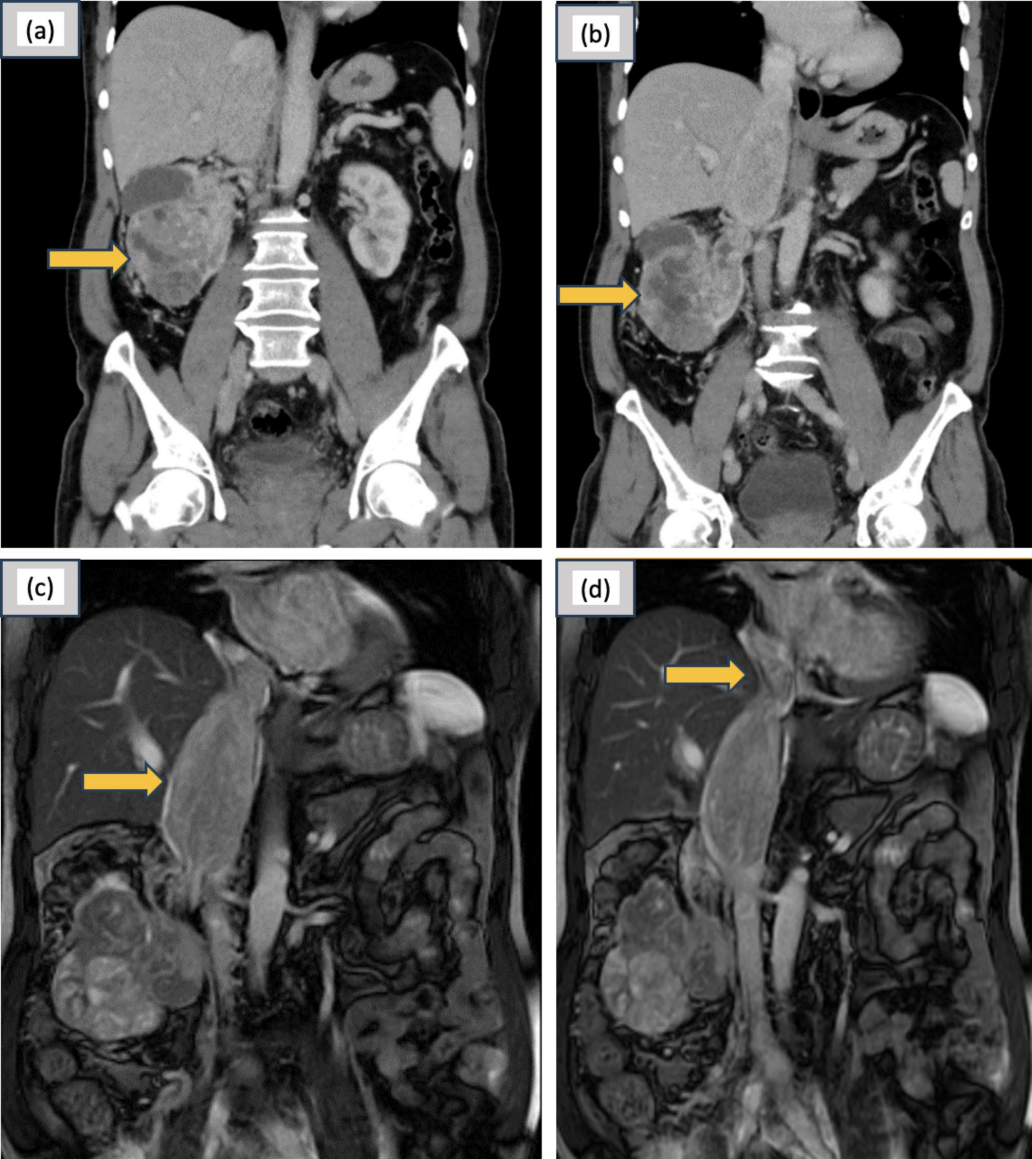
(a) & (b) CECT images showing the Enhancing Renal Mass; (c) & (d) MRI images showing the enhancing Tumour Thrombus extending upto the right atrium
